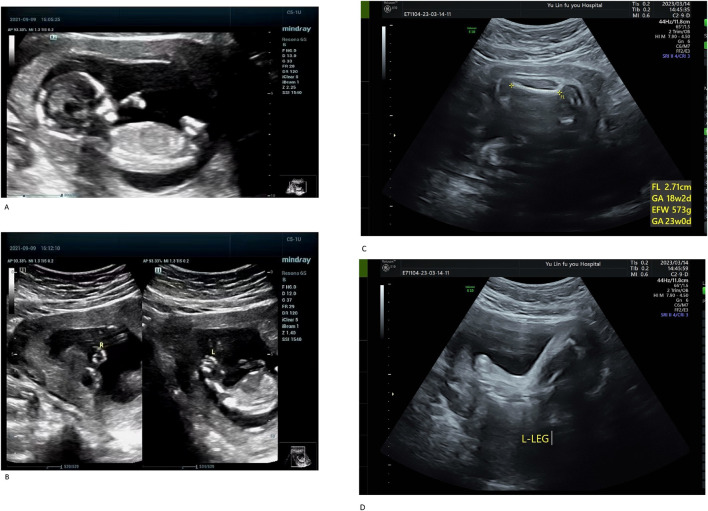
(A) Systemic fetal ultrasound results of the proband (III-1). (B) Systemic fetal ultrasound results of the proband (III-1). Ultrasound examination revealed shortened limbs, abnormal positioning of both hands (suggestive of bilateral radial aplasia), fixed posture of both lower limbs, and bilateral clubfoot. Reversed a-wave in the ductus venosus was also observed, leading to pregnancy termination (A,B). (C) Systemic fetal ultrasound results of III-3. (D) Systemic fetal ultrasound results of III-3. At 26 weeks of gestation during her third pregnancy, Case III-3 underwent a level II ultrasound, which revealed shortened limbs, bilateral clubfoot, and an enlarged gallbladder (C,D).
